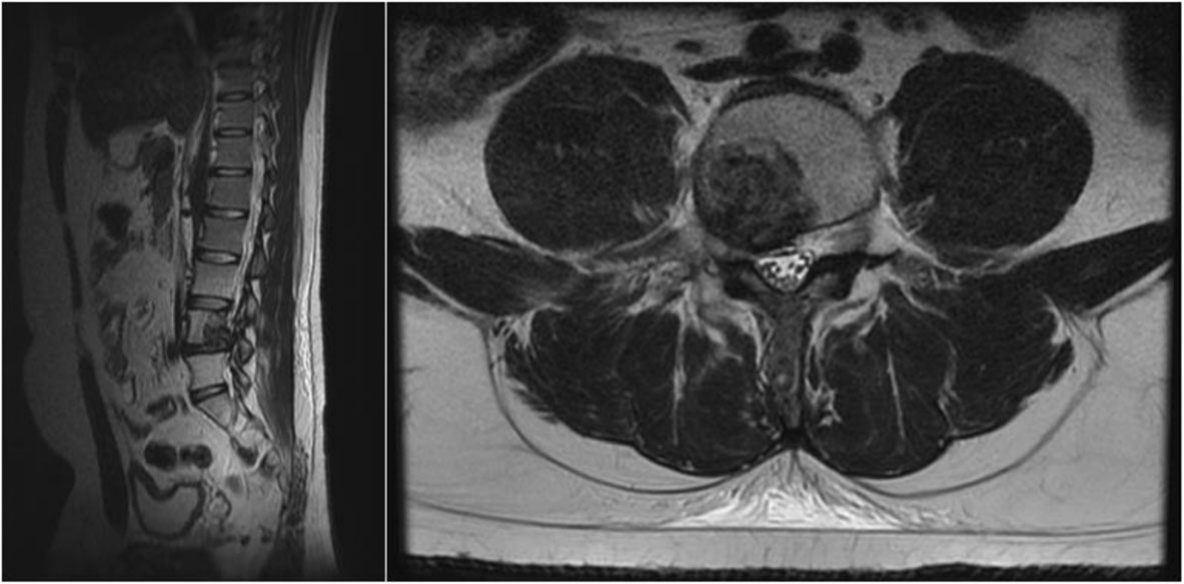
Follow-up magnetic resonance imaging at 3 months demonstrated interval improvement including post-radiation changes and decreased enhancement
